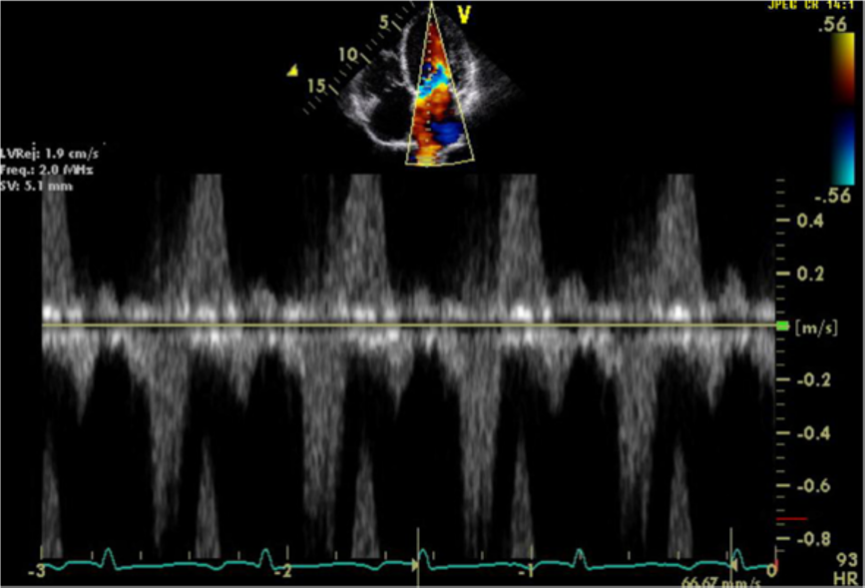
Reversal of pulmonary vein flow. Pulsed wave Doppler interrogation of the right upper pulmonary vein in this apical four chamber view shows systolic reversal of flow. This is a specific, albeit lower sensitivity, sign of severe MR.
